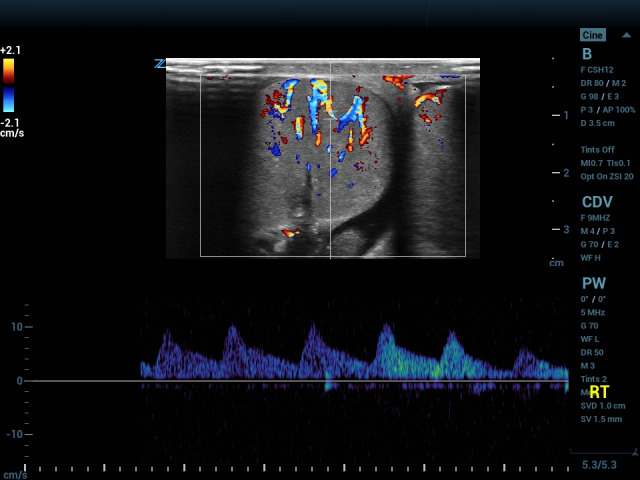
Doppler gate is placed over a blood vessel and shows atardus parvus pattern.
